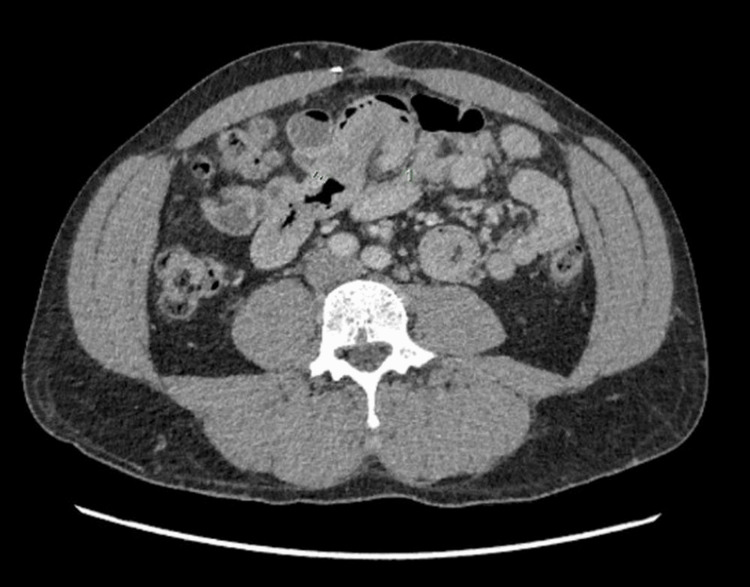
CT scan of the abdomen demonstrating an area of intussusception resulting in a mushroom sign.
